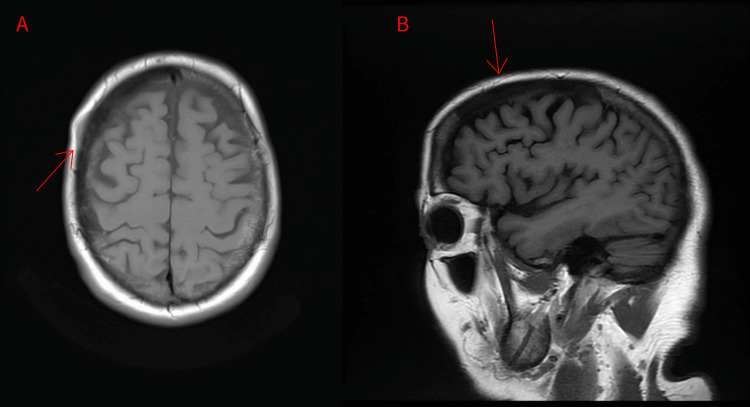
Magnetic resonance imaging showing T1 hypointensity involving the right anterior calvarium (white line) measuring 8.5 cm from the (A) axial and (B) sagittal views. There is plaque-like enhancement along the inner and outer tables associated with this lesion.
